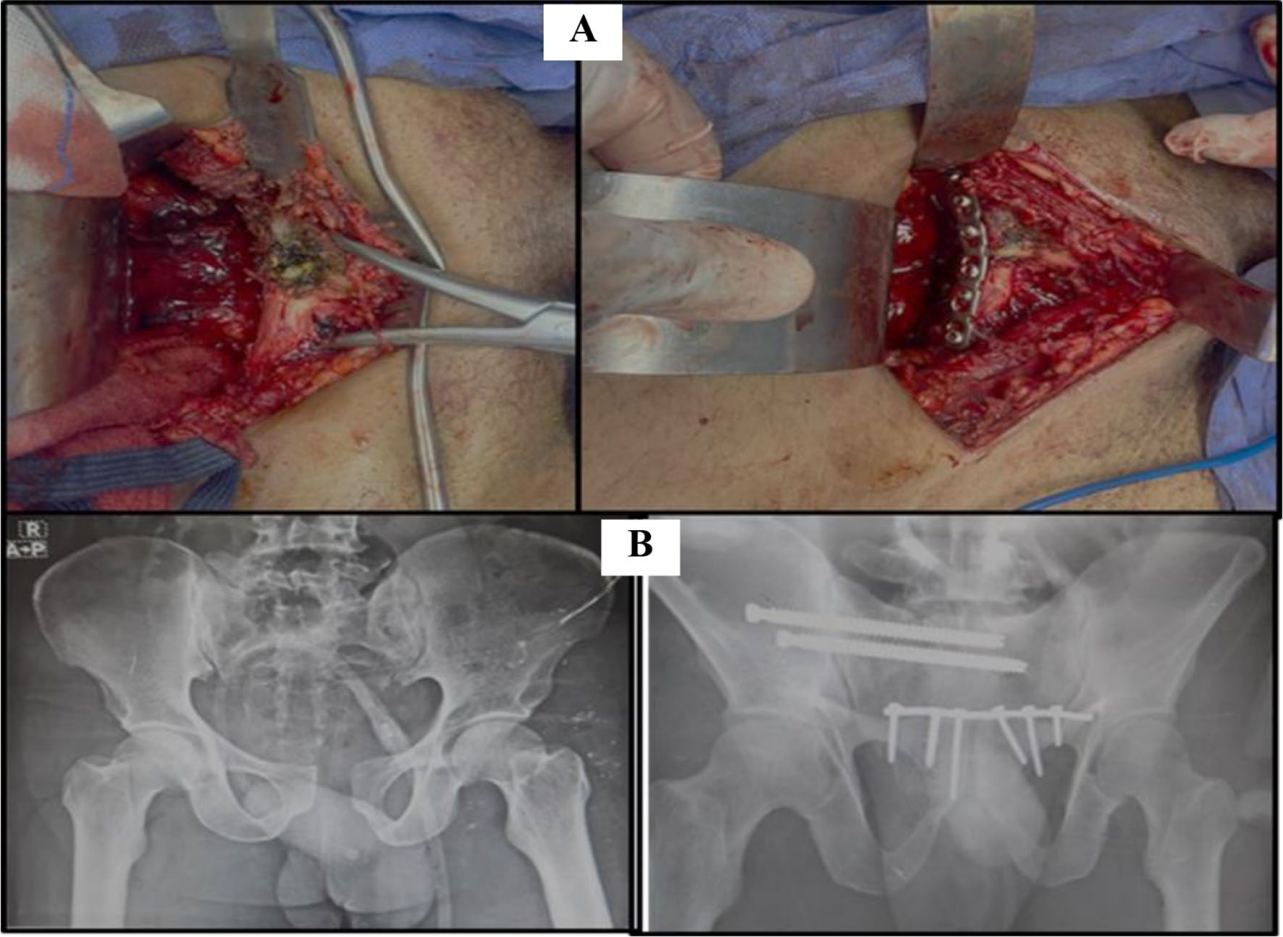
(A) Reduction of the diastasis and application of pre-bending reconstructive plate notch. (B) X-ray before and after symphyseal plating
